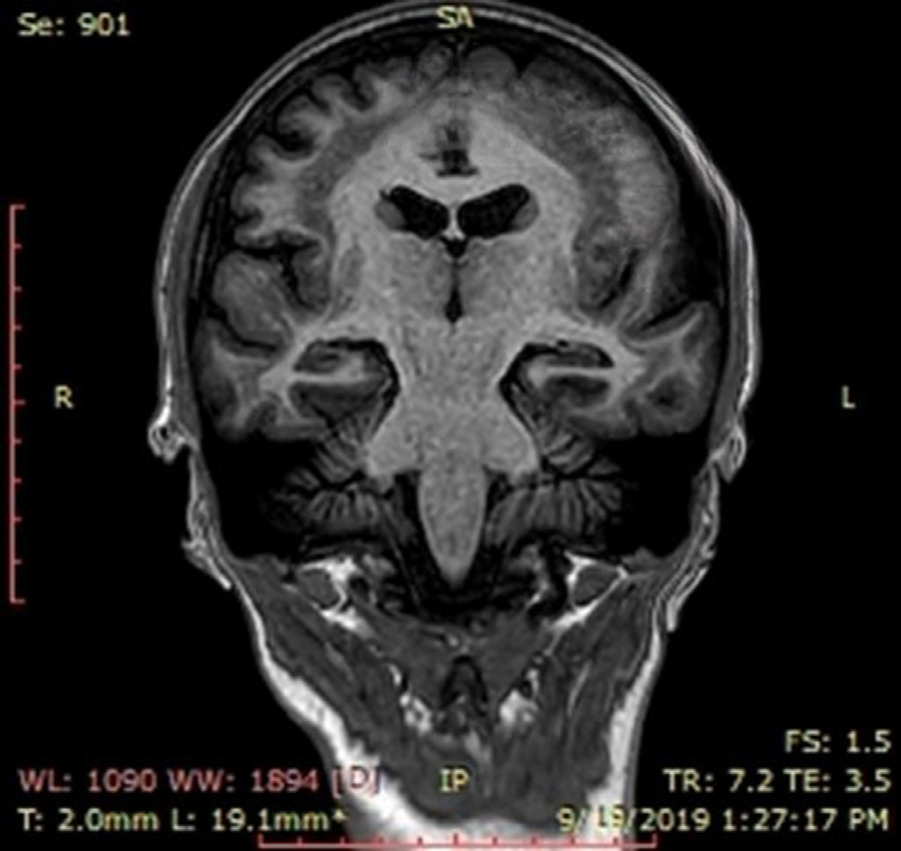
MRI brain T1WI 3D TFE Coronal images - The heterotopic grey matter almost paralleling the cortex is appreciated.
